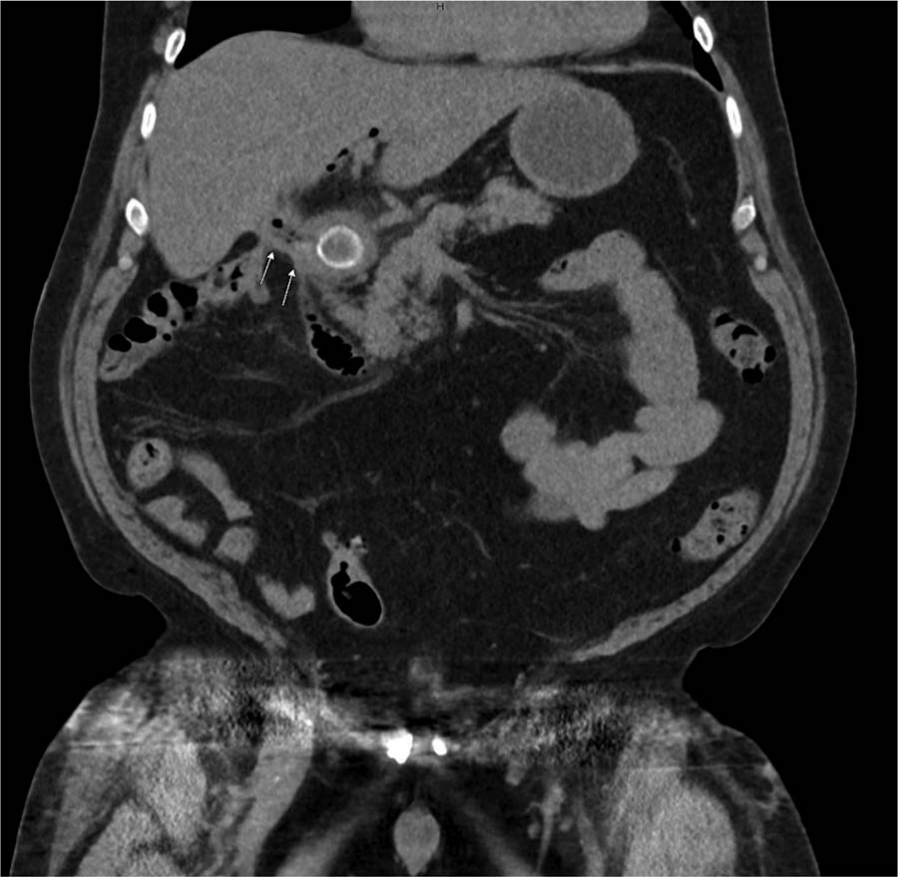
CT of the abdomen without contrast showing a large stone measuring ~3 × 2.1 cm in the proximal duodenum with surrounding induration suggesting a choledochoenteric fistula as indicated by the arrows.
